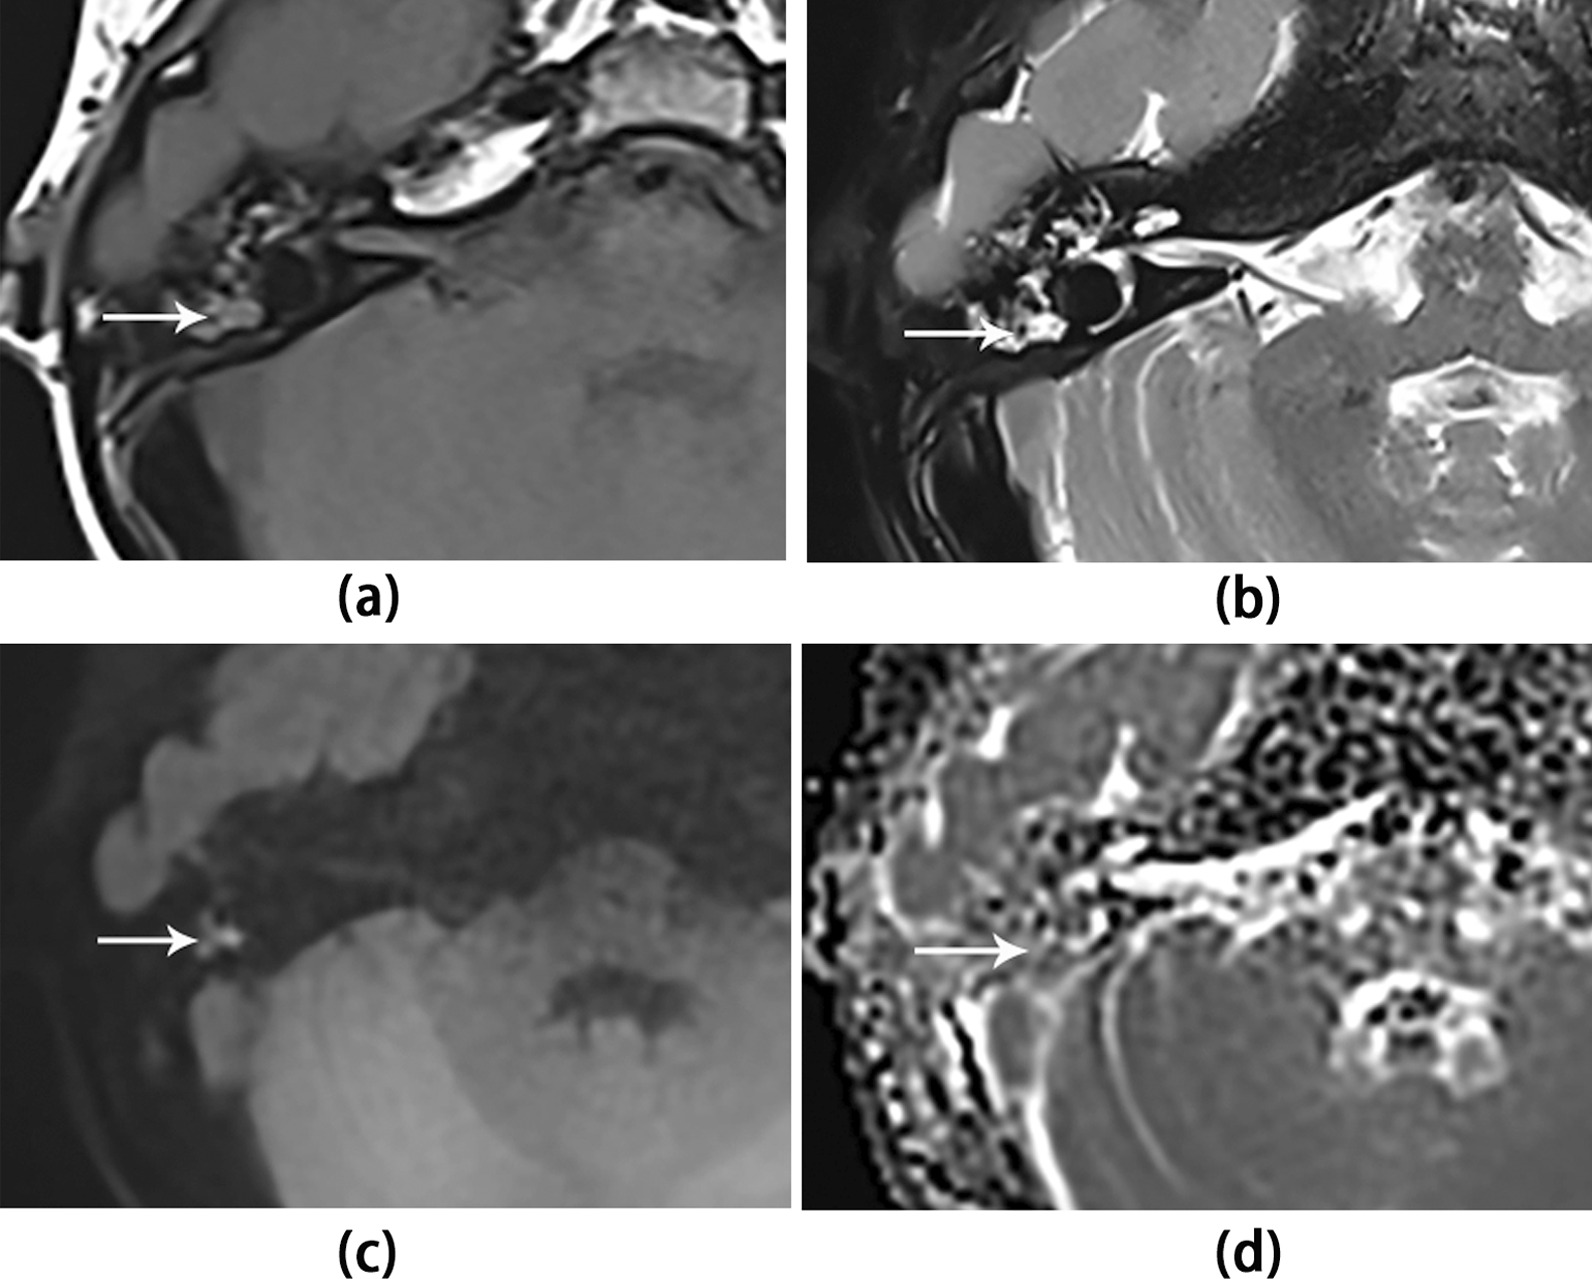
A 37-year-old male patient with false-negative NCS on the right side. Axial images show the lesion (white arrow) as low signal on (a) the T1WI and (d) ADC map, and high signal on (b) the T2WI-FS and (c) TGSE BLADE DWI b1000 image
